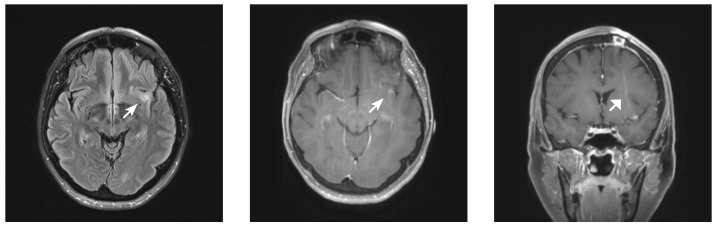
Magnetic resonance imaging demonstrating complete resolution of previously noted contrast enhancement, within a markedly reduced region of insular T2 hyperintensity (arrowheads); of note, a strip of expected T2 hyperintensity was noted surrounding the needle tract, excluding a technical error underlying the signal change at the lesion (broad arrow).
